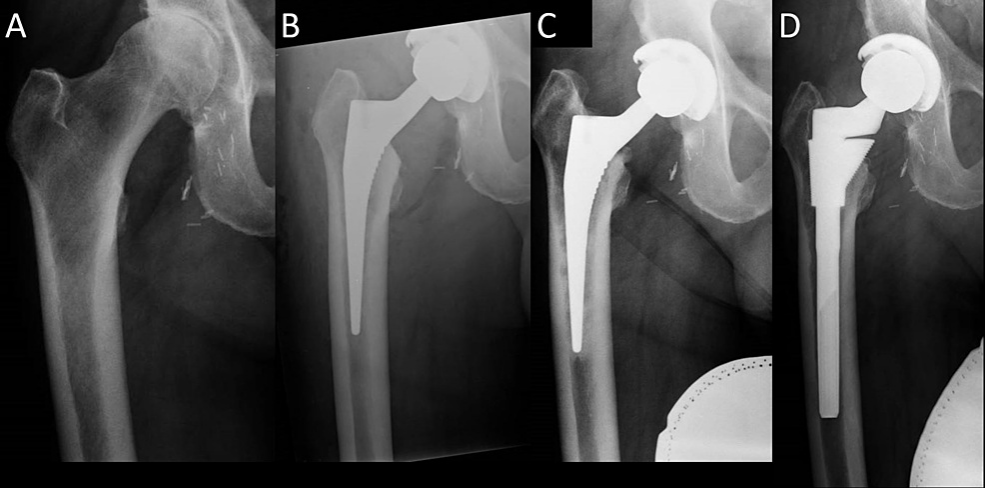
Radiographs of patient 3. (A) Preoperative AP of patient 3 with Dorr A bone and canal-flare index of 4.9. (B) Postoperative AP of Corail size 9, collared, high-offset stem with a +5.5 mm head. (C) Follow-up radiographs with subsidence and varus migration. (D) Revision of S-ROM stem. AP, anteroposterior view
